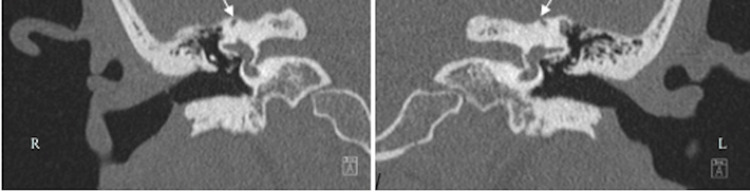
A 39-year-old woman presenting with many auditory and vestibular symptoms including hyperacusis, tinnitus, and vertigo. High-resolution CT scan shows thinning of the (R) right and (L) left osseous roof of the bilateral semicircular canals with possible areas of dehiscence. CT, computed tomography
